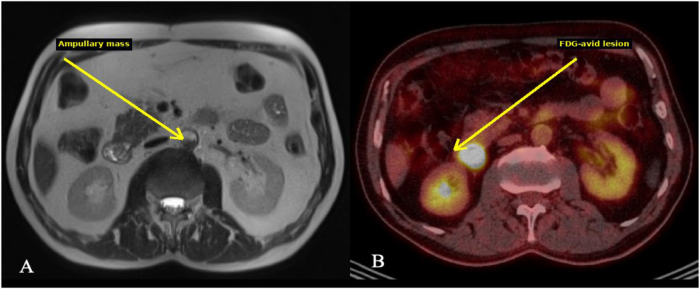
Pre-operative imaging. (A) Axial MRI demonstrating the ampullary mass (arrow) at the level of the pancreatic head, with associated biliary ductal changes. (B) PET-CT showing a focal FDG-avid lesion (arrow) at the ampulla of Vater, with no evidence of nodal or distant metastatic disease.
